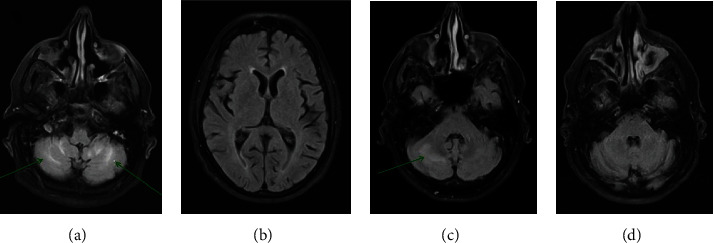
T2-FLAIR Magnetic Resonance Imaging (MRI) brain with contrast demonstrating (a) symmetric T2-hyperintensity of the cerebellum on initial case presentation, (b) representative image demonstrating lack of cerebral involvement during initial case presentation, (c) subsequent 2-week follow-up MRI demonstrating resolving T2-hyperintensity in the cerebellum, and (d) complete resolution of cerebellar T2-hyperintensity 6 months postpresentation.
